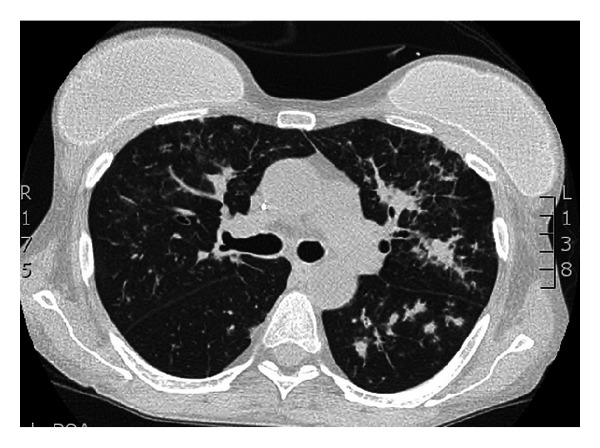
Chest CT scan showing confluent nodules and ground-glass patchy consolidations especially in the left lung.
